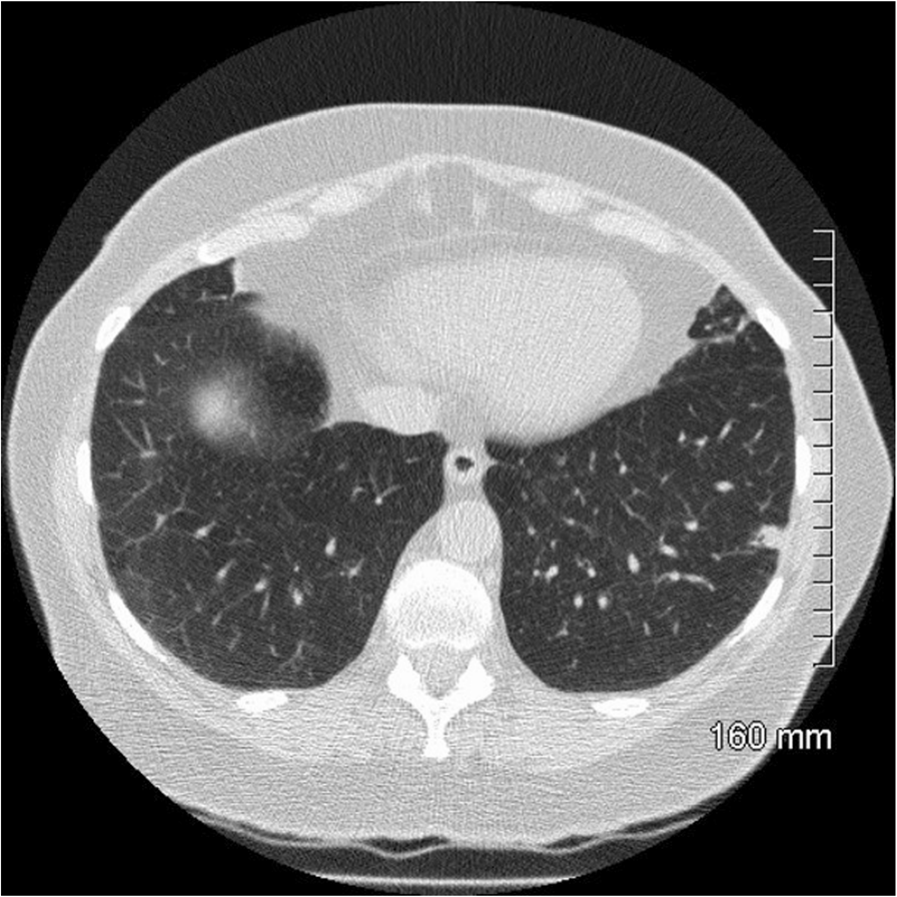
MIA on CT: 9 mm part-solid subpleural nodule, predominantly solid, in the left lower lobe
